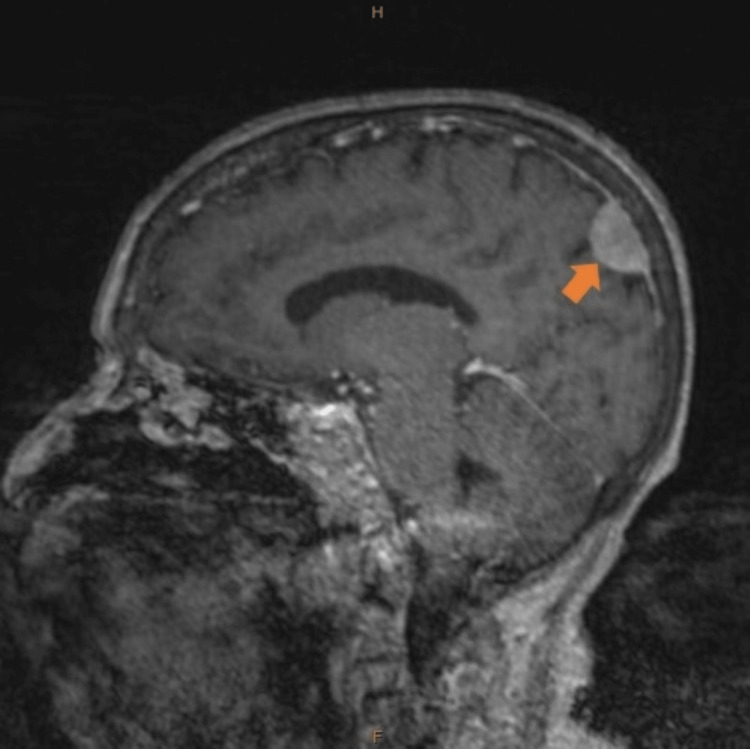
T1 sagittal magnetic resonance imaging (MRI) brain showing left parafalcine parietal lobe mass (arrow). H- head end, F- foot end.
